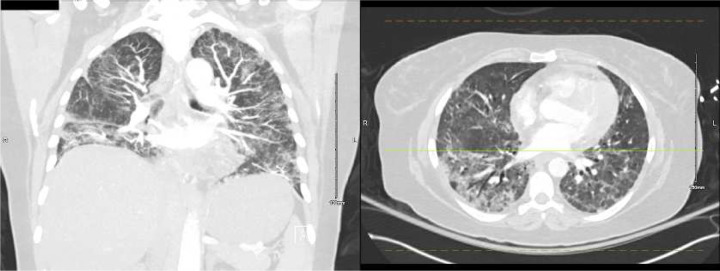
Outpatient chest computed tomography two days before the patient’s third admission to the hospital, showing ground glass and airspace opacities in the lower lungs differening in distribution from the first admission, with a few focal areas of honeycombing, most prominently in the left upper lobe.
